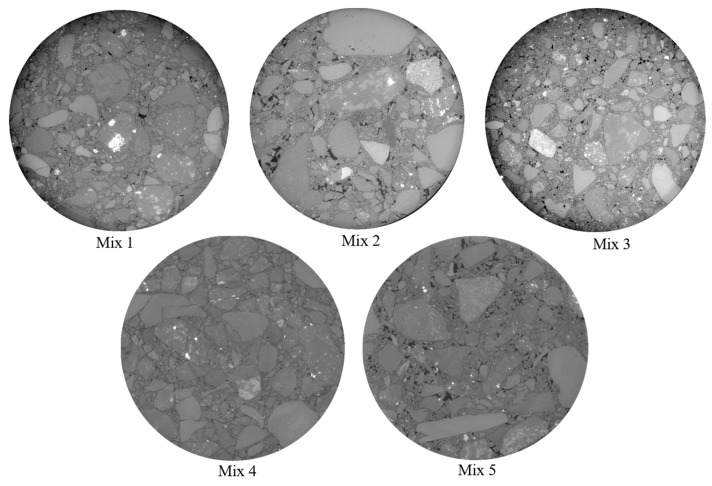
An example of 2D X-ray CT images for the tested AC mixtures at 0 F–T cycles.
